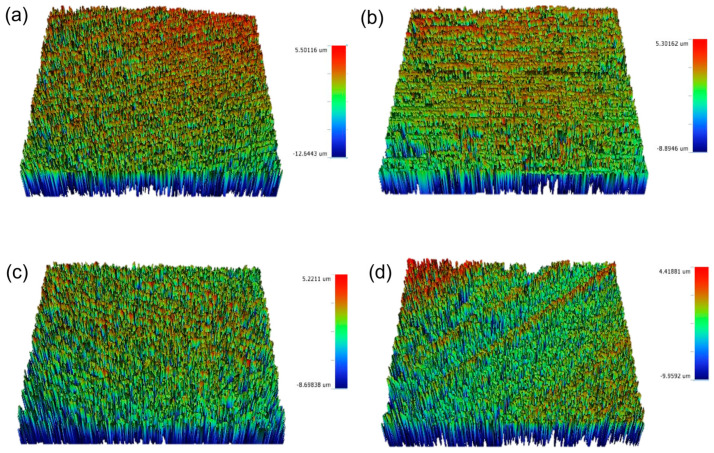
Surface roughness (Ra) of manual plastic scaling of each: (a) CD, (b) CT, (c) IPE, and (d) IPS. CD; zirconia-reinforced lithium silicate (Celtra Duo). CT; advanced lithium disilicate (CEREC Tessera). IPE; lithium disilicate (IPS Emax CAD). IPS; leucite-reinforced (IPS Empress CAD).
